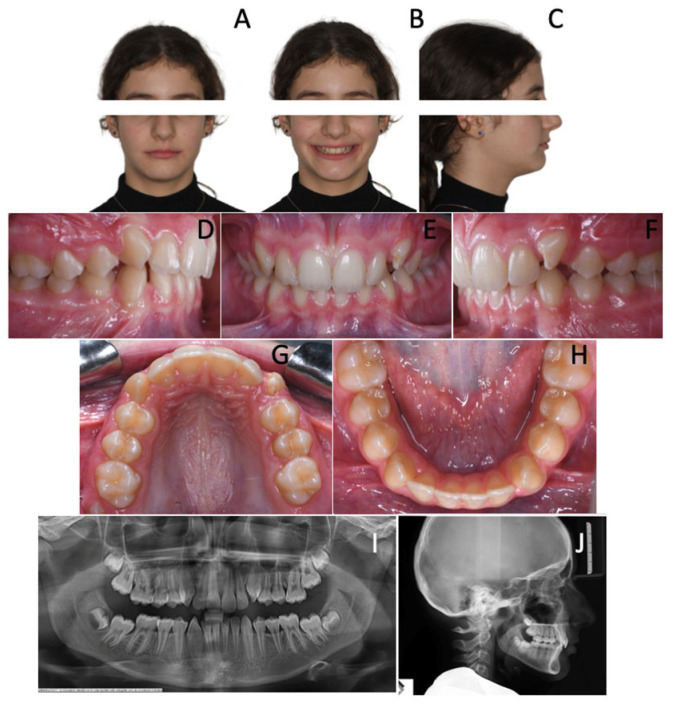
Patient at the end of phase I. (A–C) Extra-oral photographs; (D–H) Intra-oral photographs; (I) Orthopantomopraphy; (J) Teleradiography.
